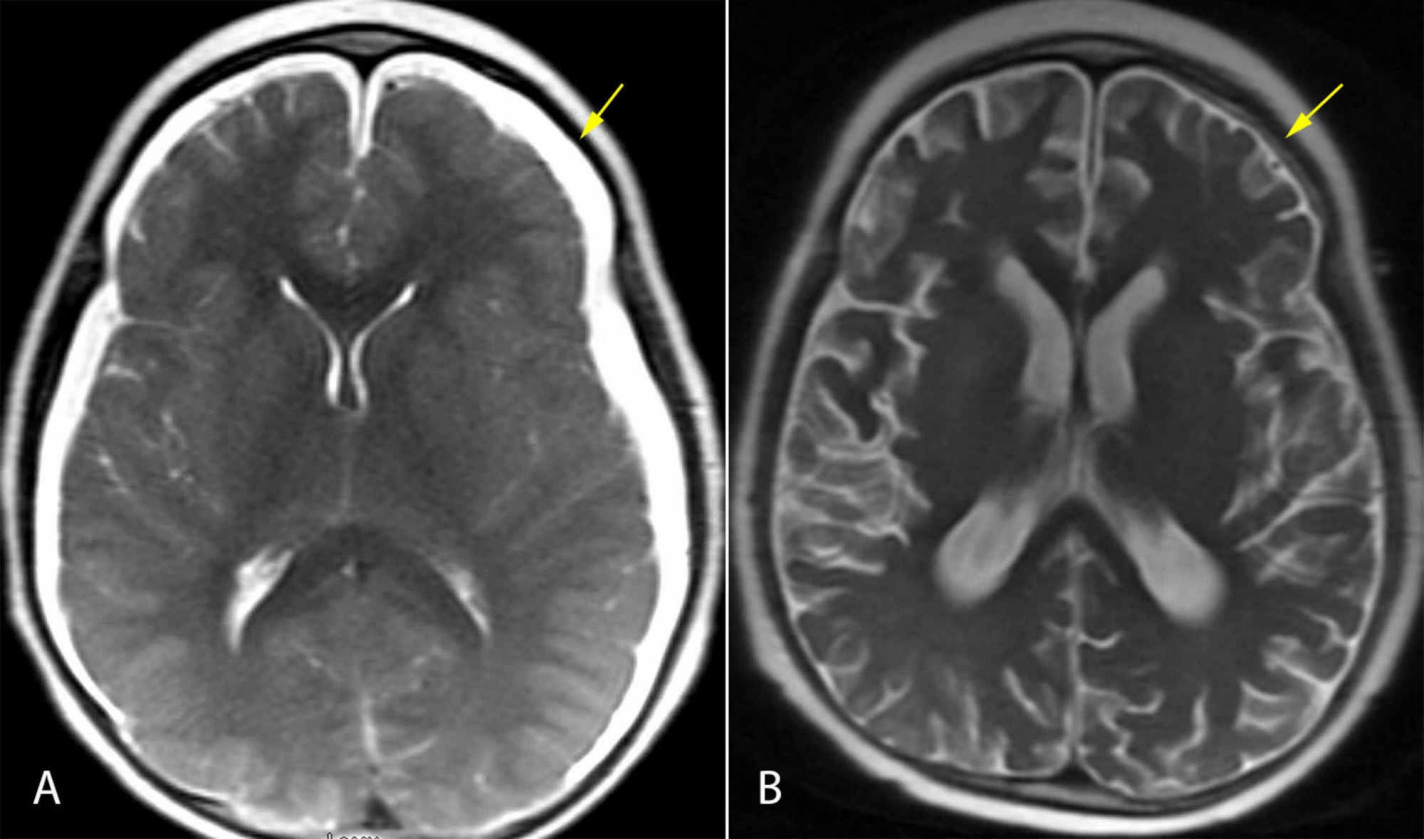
Case 1 Initial brain MR images demonstrating subdural fluid collections, ventricular compression, and caudal descent of the brainstem (A). These findings reversed postoperatively (B).
